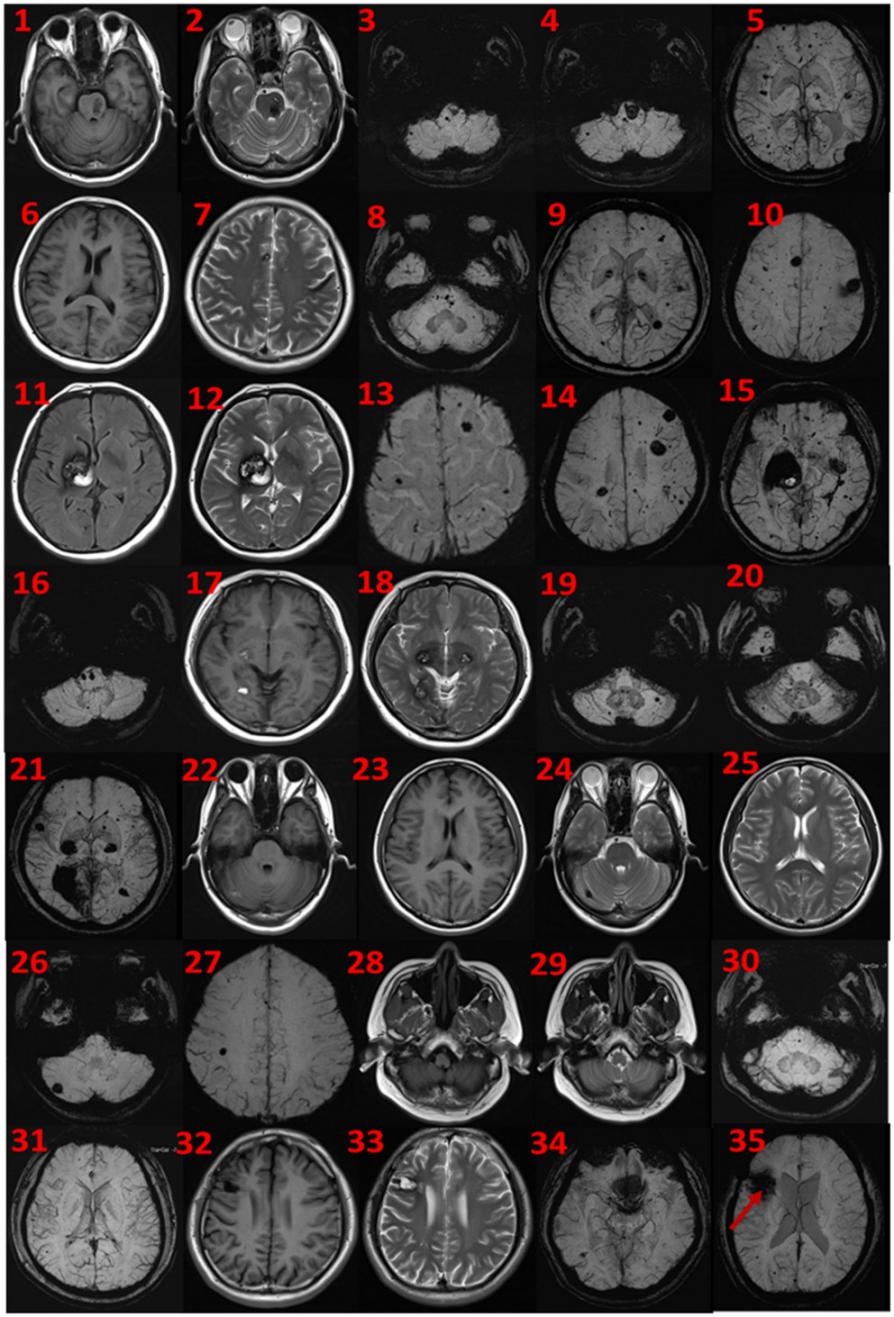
MRI findings. Cerebral MRI in subjects II-1 (1–5), II-4 (6–10), II-6 (11–16), II-8 (17–21), III-2 (22–27), III-3 (28–31), and iii-4 (32–35). 1–5 the MRI imaging showing there are multiple lesions in bilateral cerebellar hemispheres, brainstem area, left thalamus, and white matter areas in both cerebral hemispheres. 6–10 the MRI imaging showing there are multiple lesions in the brain stem and bilateral cerebral hemispheres. 11–16 the MRI imaging showing there are multiple lesions in the cerebellum, brainstem, and bilateral cerebral hemispheres, the large lesions are located in the right basal ganglia area, with a slight space–occupying effect. 17–21 the MRI imaging showing there are multiple lesions in bilateral cerebellar hemispheres, brainstem, bilateral thalamus, and bilateral cerebral hemispheres. 22–27 the MRI imaging showing there are lesions in the right cerebellar hemisphere and the right parietal lobe. 28–31 the MRI imaging showing there are multiple lesions on both sides of the brain, and the arrow points to the postoperative manifestations. 33–35 the MRI imaging showing there are multiple lesions in the brain stem and bilateral cerebral hemispheres.
